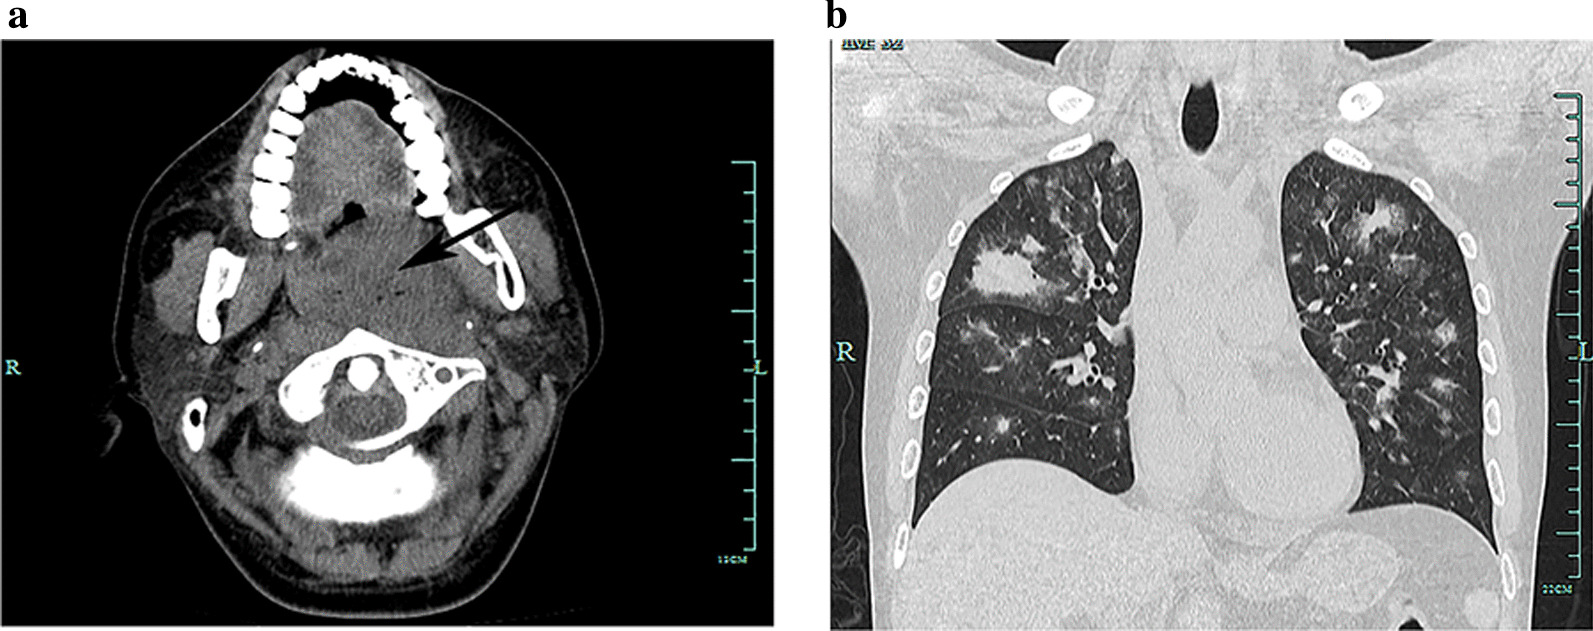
CT images of the neck and the chest on admission. a The arrow shows an oval soft tissue mass was in the oropharyngeal cavity, about 3 cm in diameter, with some gas shadows in the lesion. b Chest CT revealed multiple lesions among both lungs. Most of them appeared as ground-glass subsolid nodules, some showed consolidation, and the lesion in the right upper lobe showed a mass-like appearance
